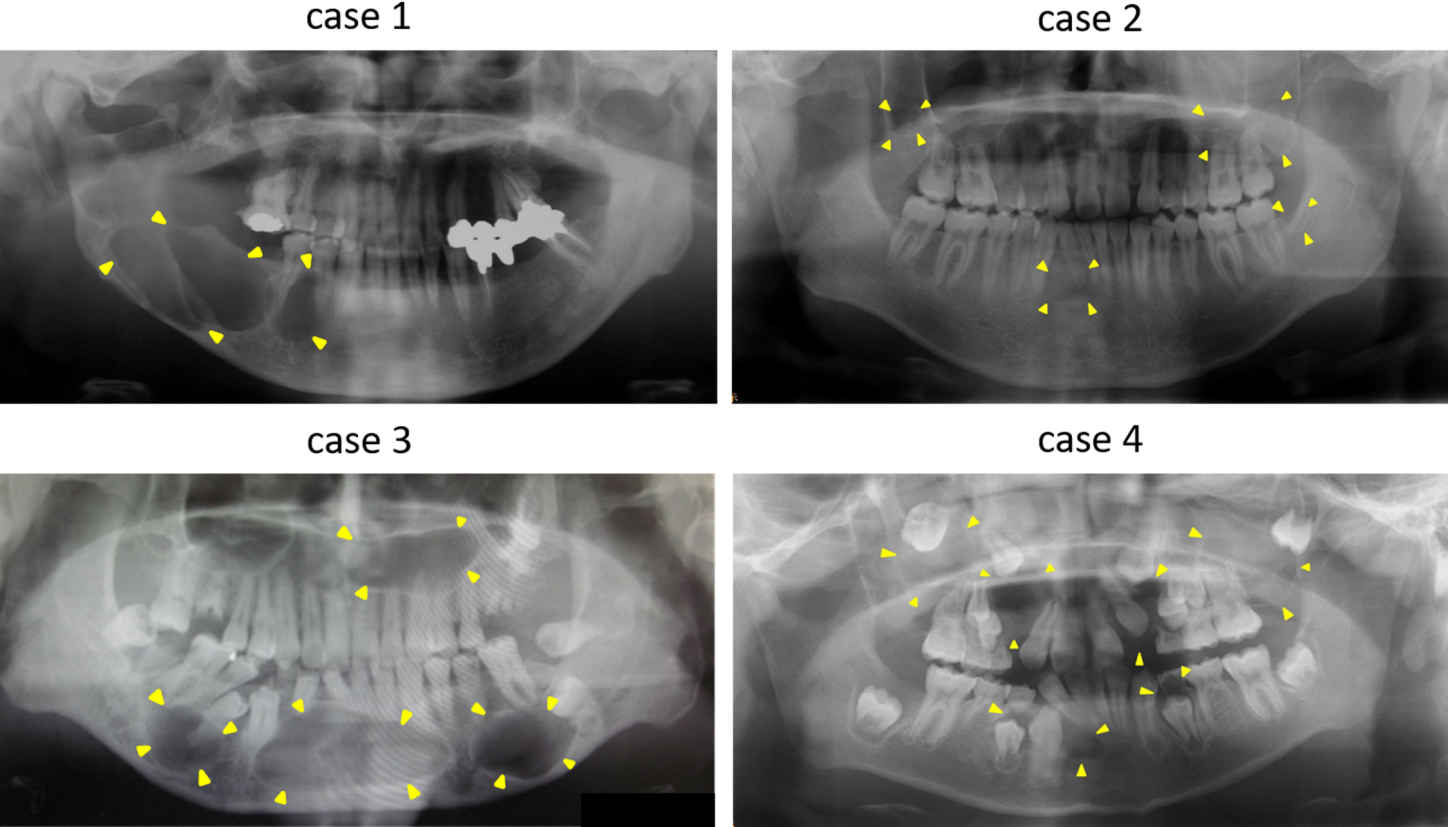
Panoramic radiograph demonstrates the presence of odontgenic keratocysts. Radiologic images showed odontogenic keratocystes in each individual. Yellow arrows showed radiolucent lesions.
